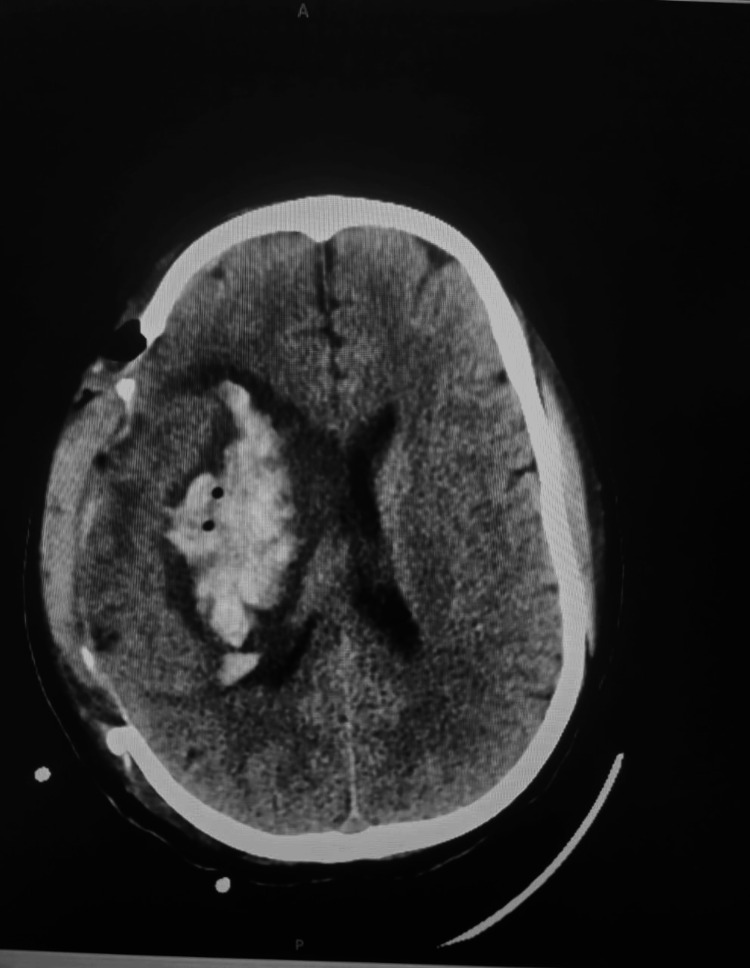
Follow-up CT of the brain on day 3 post-craniectomy
